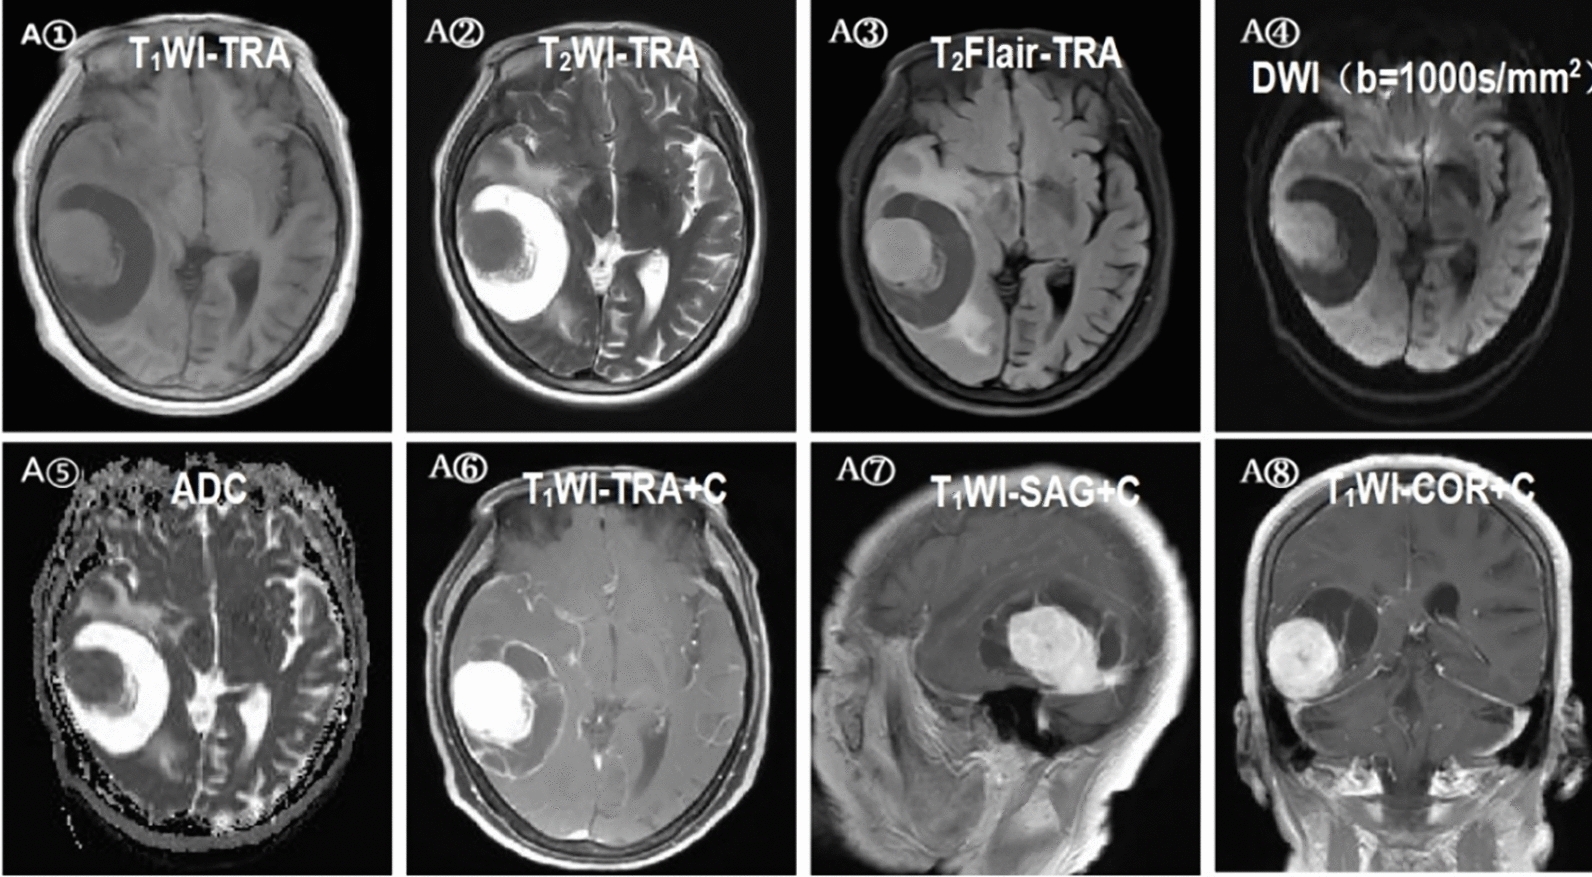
A①–⑧ A 77 year-old woman with GII ISFT. MRI shows a cystic-solid mass with isohomogeneous intensity on the T1WI, T2WI, and T2-FLAIR images (A①–③), slight hyperintensity on the DWI images (*b* value = 1000 s/mm^2^) (A④), slight hypointensity on the ADC maps (A⑤), and slight inhomogeneous enhancement on the contrast-enhanced images (A⑥–⑧). *T1WI* T1‑weighted imaging, *T2WI* T2‑weighted imaging, *FLAIR* fluid-attenuated inversion recovery, *DWI* diffusion‑weighted imaging, *ADC* apparent diffusion coefficient, *TRA* transverse view, *SAG*-sagittal, *COR* coronal
